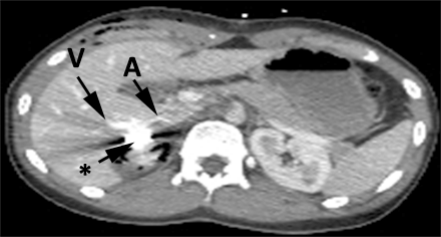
Abdominal CT. Black arrow –metallic fragment. A – replacing right hepatic artery branch (branching off the superior mesenteric artery). Ⅴ – portal branch. Arteriovenous fistula compatible aspect.
